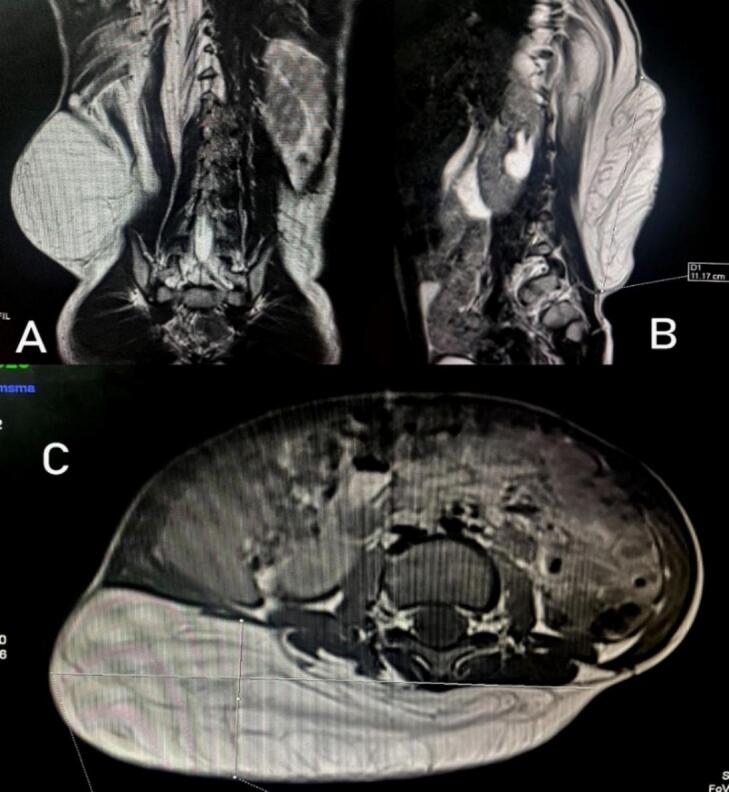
MRI findings of right dorso lumbar lipoma.
